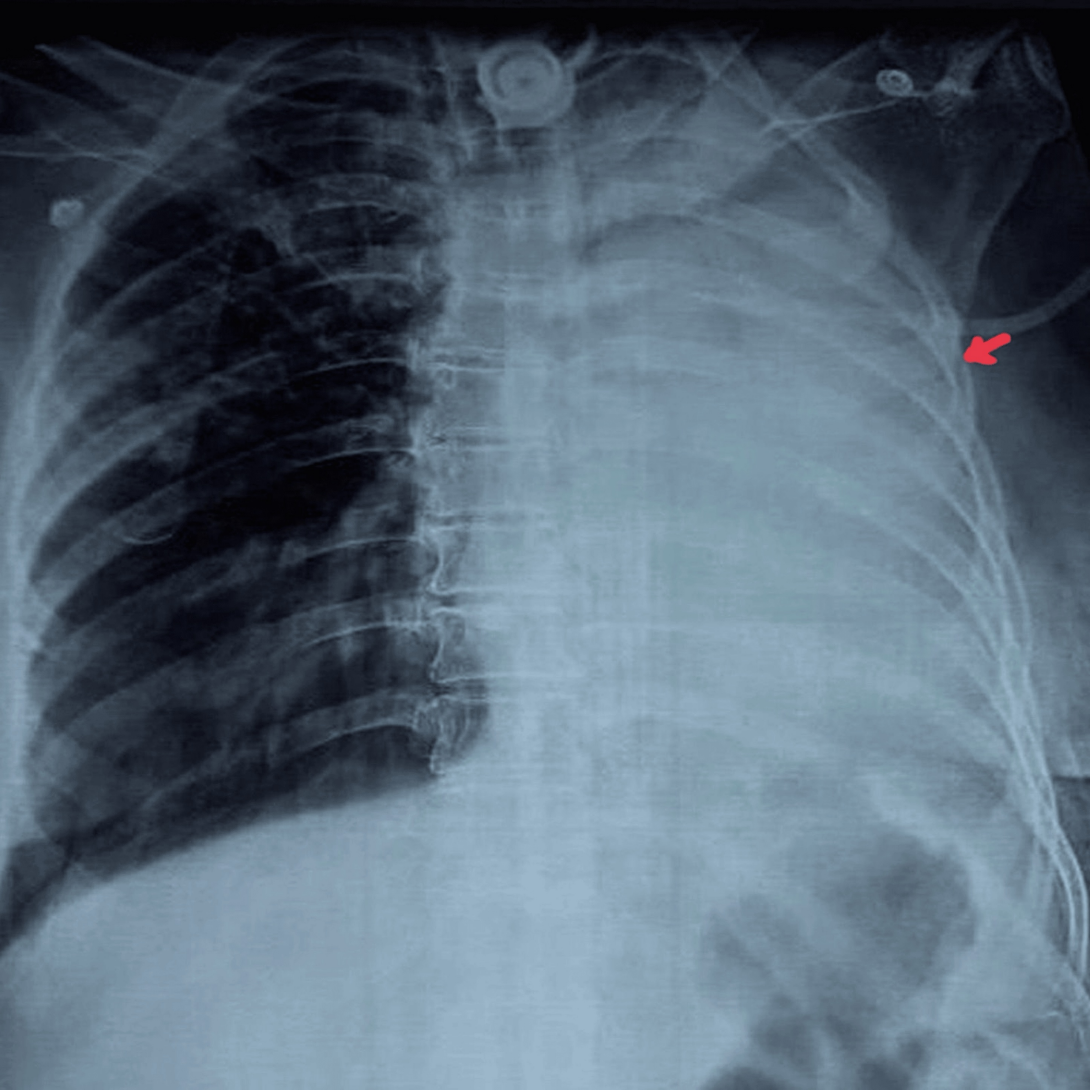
Chest X-ray showing collapse of the left lung (red arrow).
